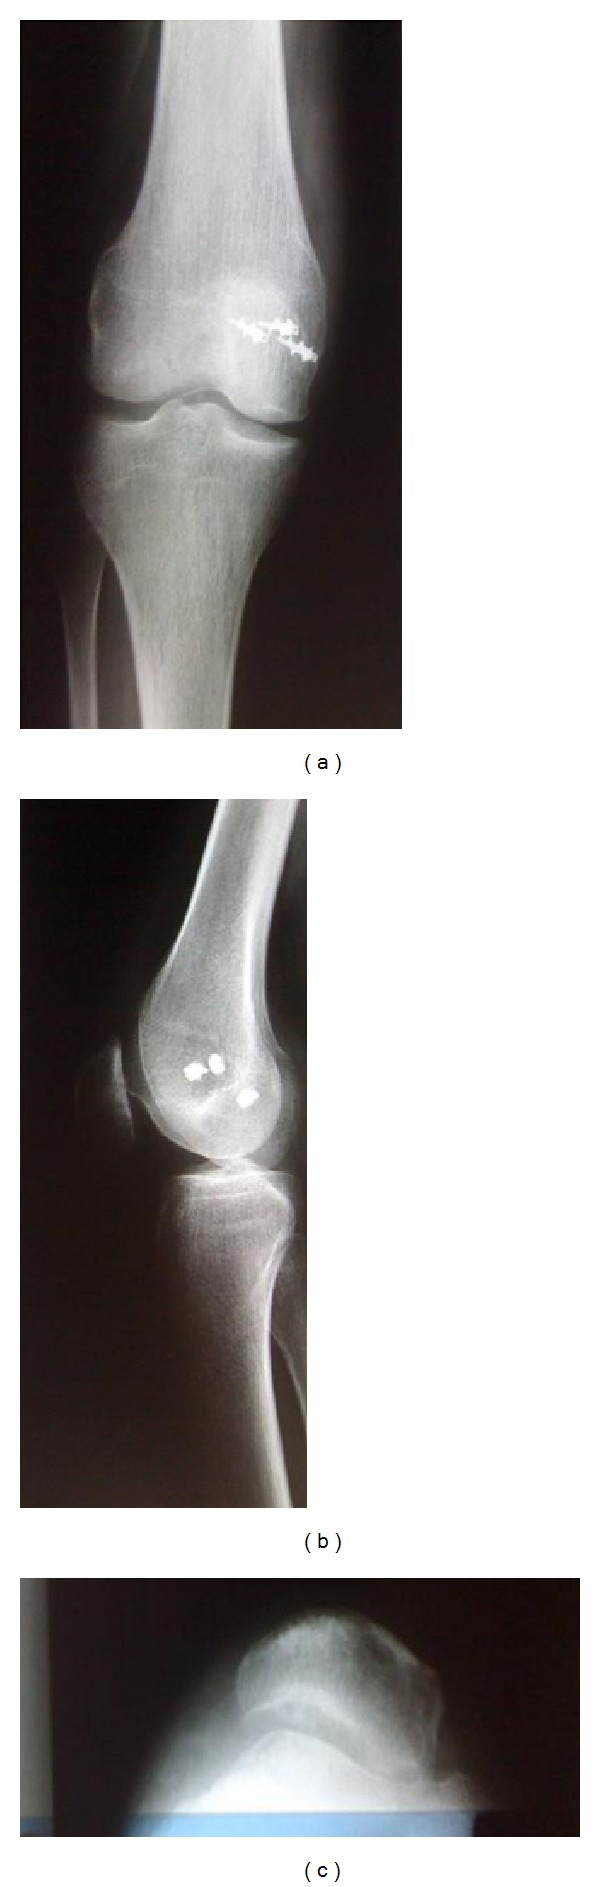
Postoperative X-rays of the knee: (a) anteroposterior, (b) lateral, and (c) tangential patellofemoral views at 60°.
